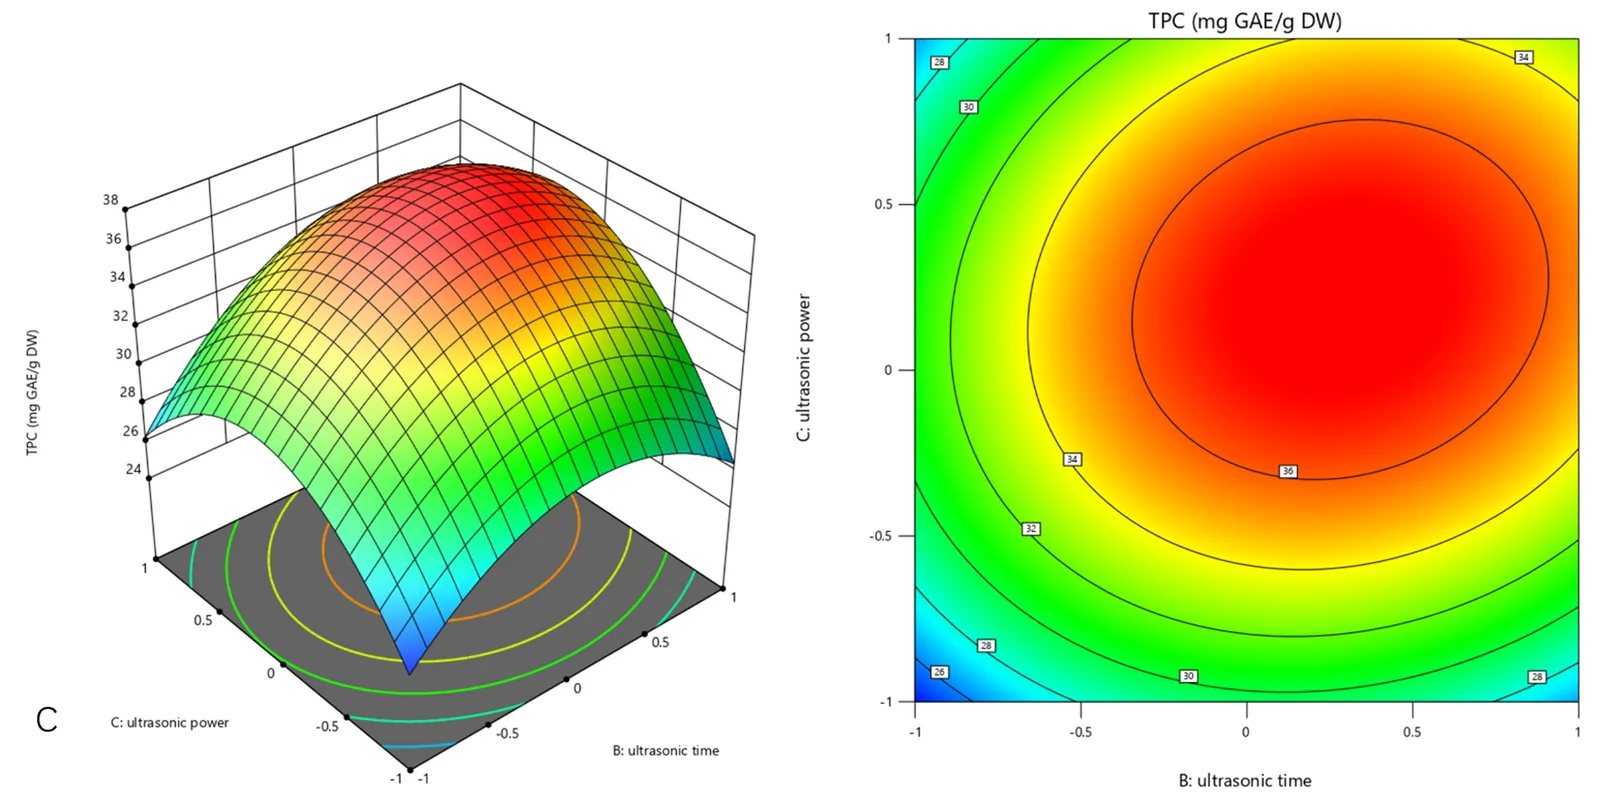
Response surface plots and contour maps showing the influence of each factor on the total polyphenol content. Note: (A) solid-to-liquid ratio and ultrasonic time; (B) solid-to-liquid ratio and ultrasonic power; (C) ultrasonic time and ultrasonic power.
